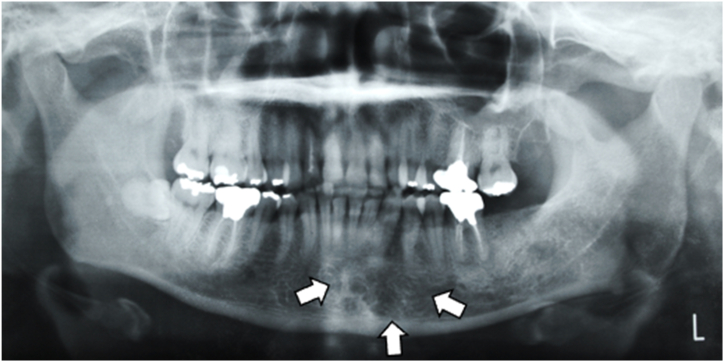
Orthopantomogram of the left side of the mandible. A mixed radiolucent–radiopaque lesion is visible.
